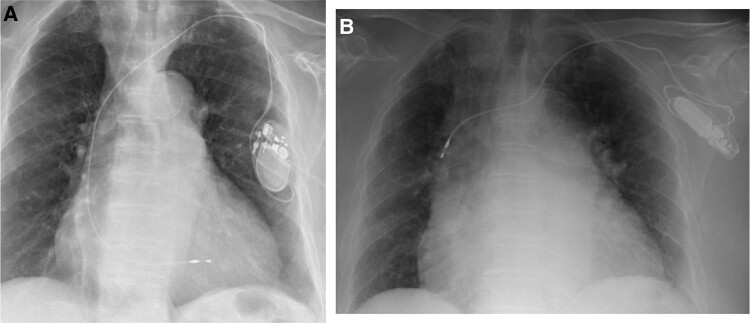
Case report 1. (*A*) Pre-discharged chest X-ray—after revision of the pacemaker and repositioning of the lead. (*B*) Chest X-ray—1 month after the pacemaker revision.
